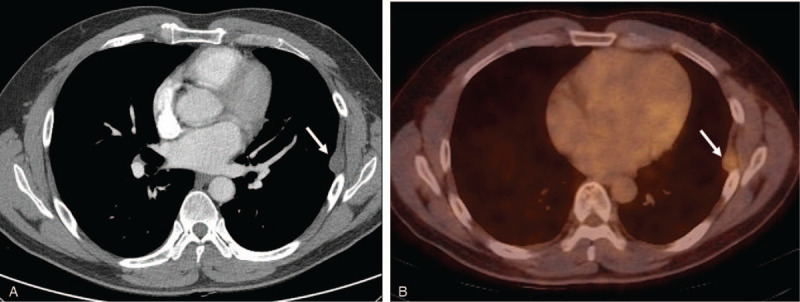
Chest computed tomography (CT) and ^18^F-fluorodeoxyglucose positron emission tomography-computed tomography (PET/CT). (A) Chest CT at a 1-year routine follow-up after left upper lobe lingular segmentectomy for T1bN0M0 lung adenocarcinoma revealed a well-circumscribed, 34-mm diameter solid tumor (arrow) attached to the left pleura, which was the prior thoracotomy site for lung cancer. (B) PET/CT showed mildly increased metabolism with a maximal standardized uptake value of 2.7 within the tumor (arrow).
